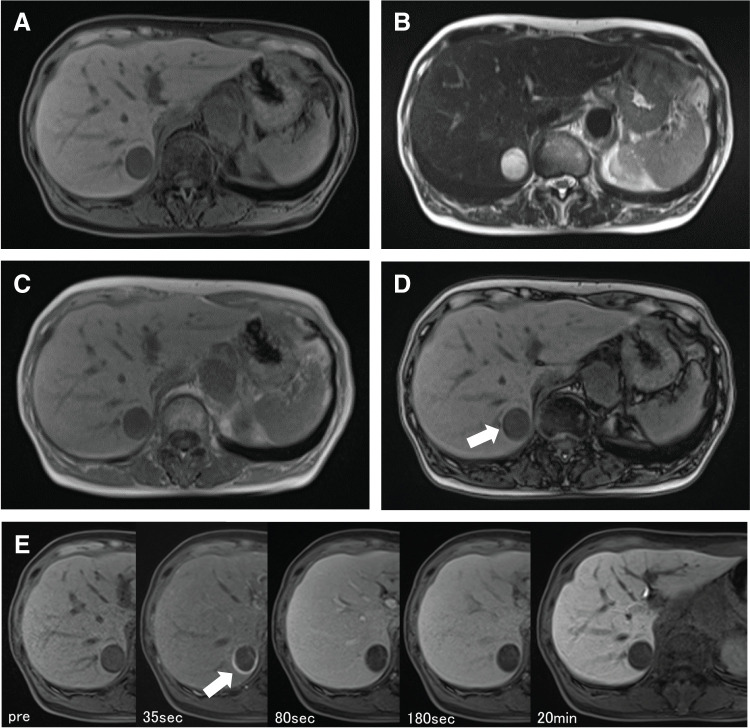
Abdominal MRI findings on admission before surgery. MRI findings showed (A) a low T1 signal and (B) a high T2 signal tumor, and (C, D) T1 in-phase and out-of-phase findings did not indicate fat throughout the tumor, although a slight signal drop was observed at the tumor margin (arrow). (E) A contrast-enhanced MRI also demonstrated a ring-like contrast enhancement in the arterial phase (arrow), but with an oligemic interior.
